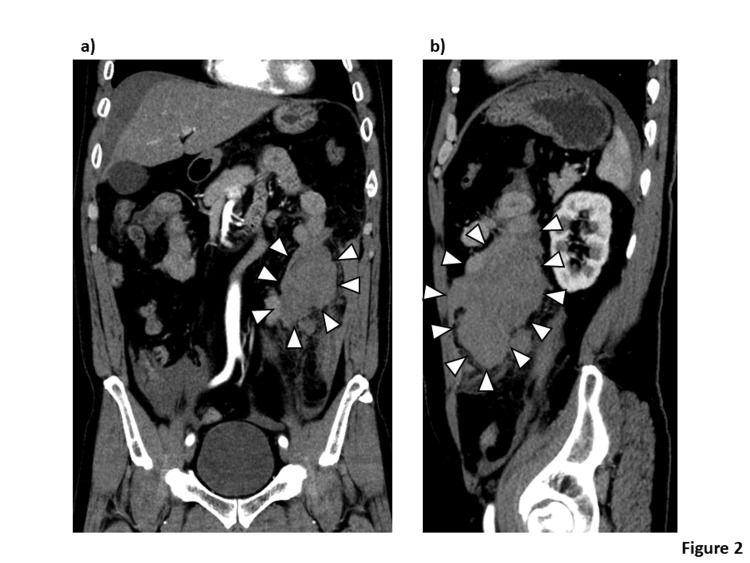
Contrast-enhanced computed tomography from the chest to pelvis: (a) coronal section, (b) sagittal section A large mesenteric hematoma (arrowhead) and intra-abdominal hemorrhage are present, without extravasation of the contrast media. The absence of active contrast extravasation indicates no ongoing arterial hemorrhage, supporting the conclusion that the volume of blood loss alone was insufficient to account for the severity of hemodynamic compromise. The large mesenteric hematoma is the proposed source of sustained mesenteric stretching and vagal activation.
